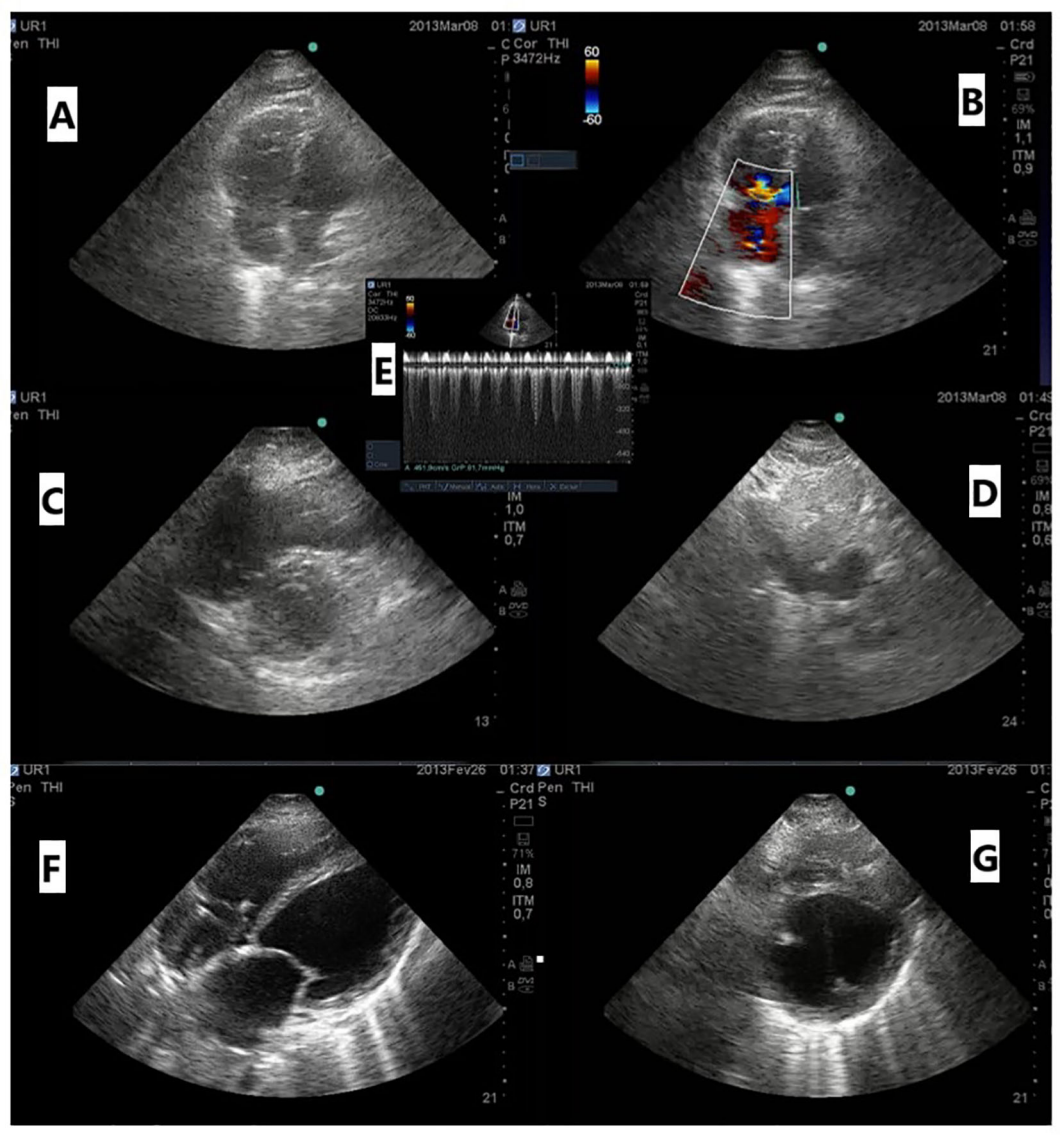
(A) Apical four-chamber (A4C) view with a dilated right ventricle (RV); (B) color Doppler of tricuspid regurgitation A4C; (C) parasternal short-axis view (PSA) showing an enlarged RV with a flattened interventricular septum; (D) dilated inferior vena cava; (E) continuous wave Doppler of tricuspid regurgitation showing estimated pulmonary systolic pressure of 96 mmHg in a patient with acute pulmonary embolism; (F) subcostal view with dilated left chambers; (G) PSA showing an enlarged left ventricle with severe systolic dysfunction related to myocarditis.
